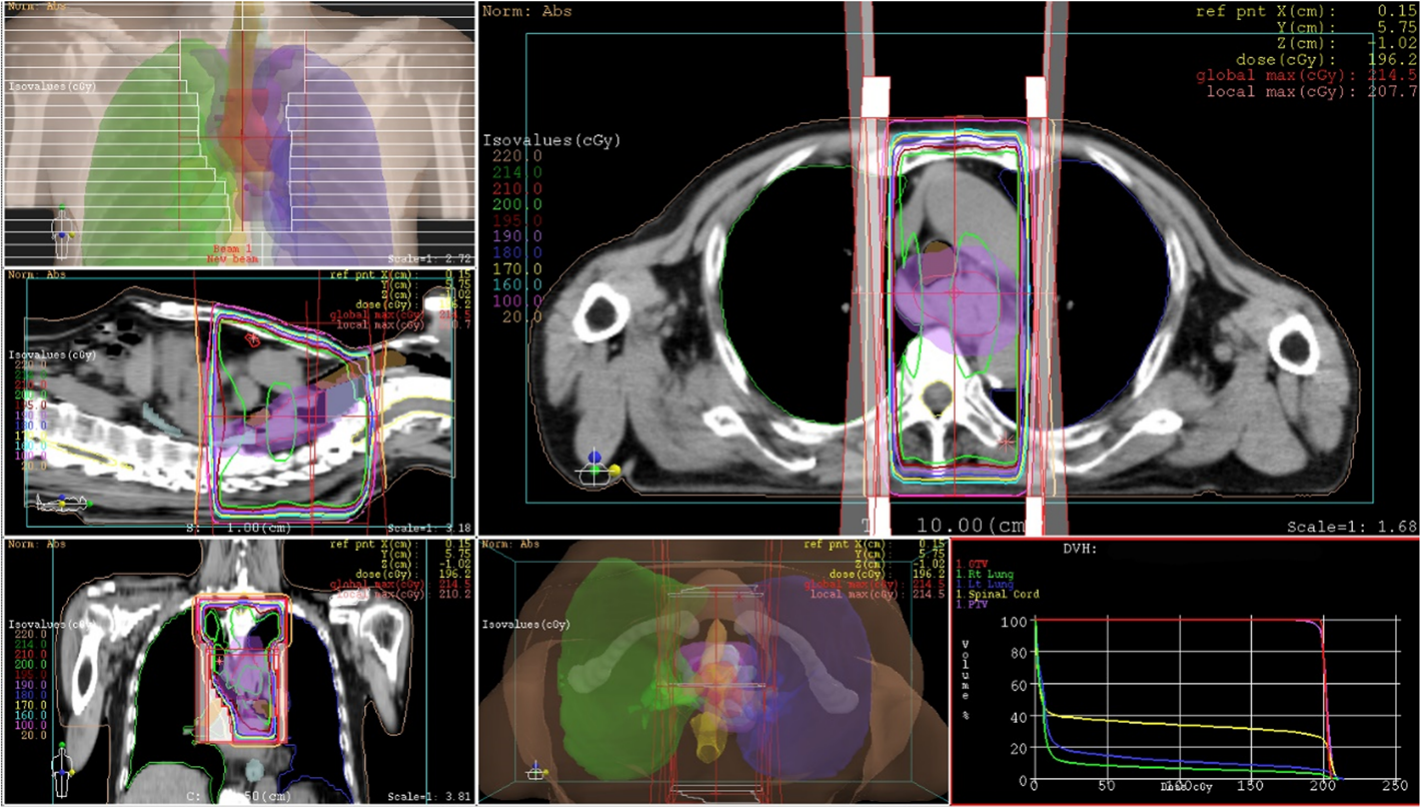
The irradiated fields and dose distributions for advanced esophageal basaloid squamous cell carcinoma irradiation using 10MV X-rays. He was prescribed a dose of 60 Gy in 2-Gy fractions, allocated as 40 Gy to the regional field and 20 Gy to the boost field. (holding the spinal cord dose below 40Gy)
